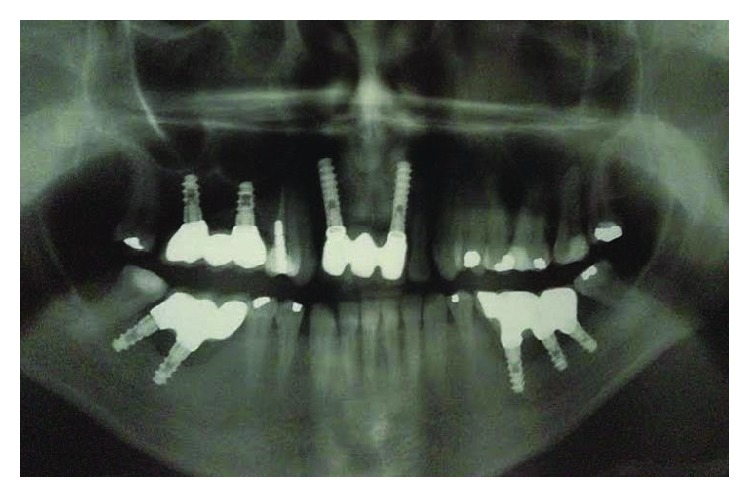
Panoramic radiography. Source: DOCEO-SC.
